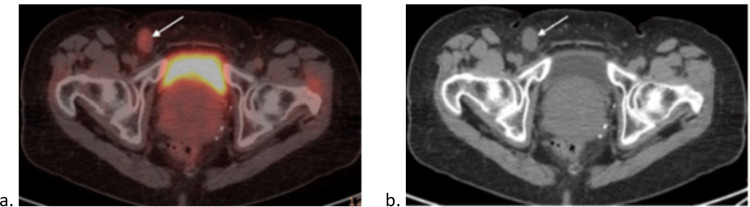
(a) PET Scan and (b) CT scan demonstrating a hypermetabolic right inguinal node (white arrows)
